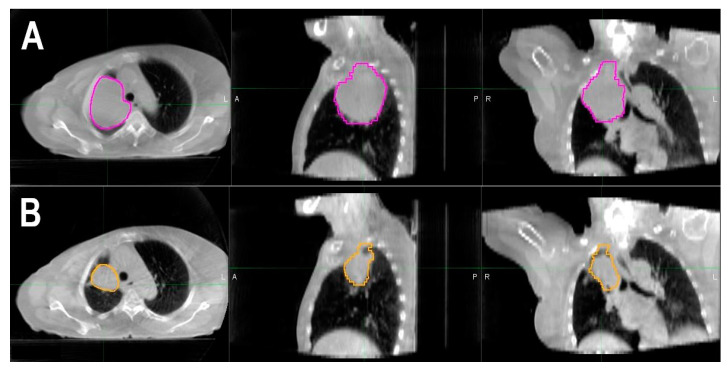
Example of gross tumor volume (GTV) contouring to determine tumor regression during chemoradiation (left to right: axial, sagittal, and coronal views). (A) Cone-beam computed tomography (CBCT) of a patient in week 1 of treatment, with GTV contoured in magenta. (B) CBCT of the patient in week 6 of treatment, with GTV contoured in gold.
